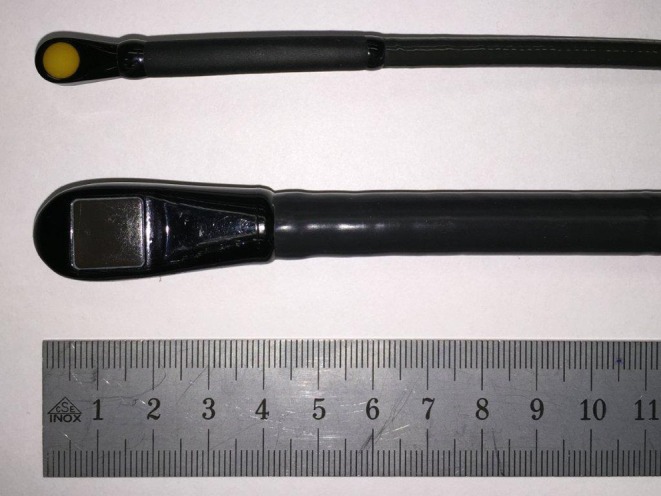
Transoesophageal probes. The S8–3t micro transoesophageal echocardiography probe (Philips Medical Systems, Andover, MA) (above) and the 3DTEE probe (X7–2t, Philips Medical Systems)
